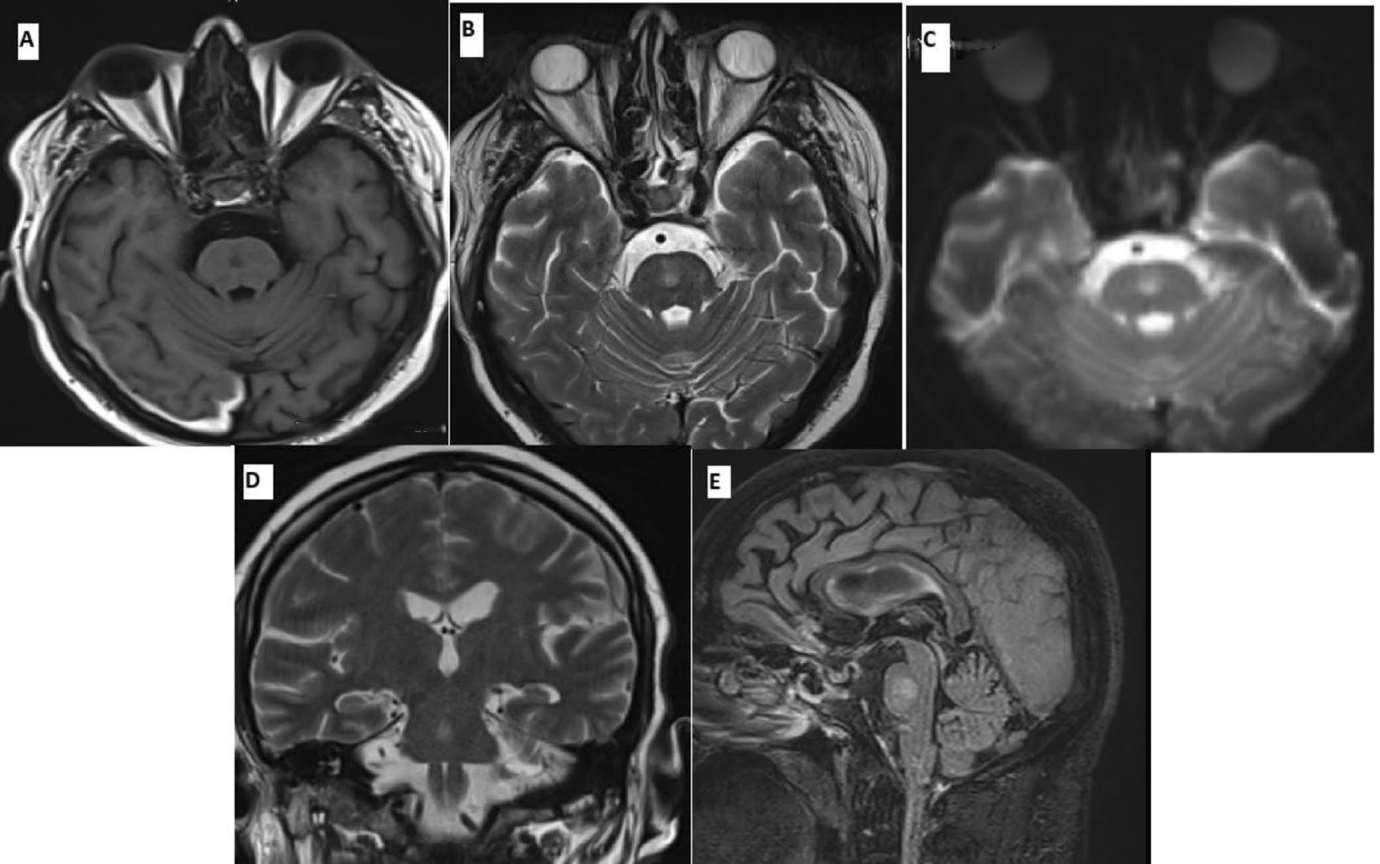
Magnetic resonance imaging (MRI) of the brain demonstrating an ovoid, slightly asymmetric lesion in the central pons. The lesion appeared hypointense on T1‐weighted images (A), hyperintense on T2‐weighted (B, D) and FLAIR images (E), and showed moderate brightness on diffusion‐weighted imaging (DWI) (C). Post‐contrast imaging revealed no gadolinium enhancement. The lesion spared the ventrolateral pons and possibly the corticospinal tracts. These findings are consistent with osmotic demyelination syndrome involving the pons, rather than an acute infarct. Additionally, there is a focal subdural hematoma measuring 8.7 mm in thickness along the right parietal convexity, which appears hyperintense on T1‐weighted images (A).
